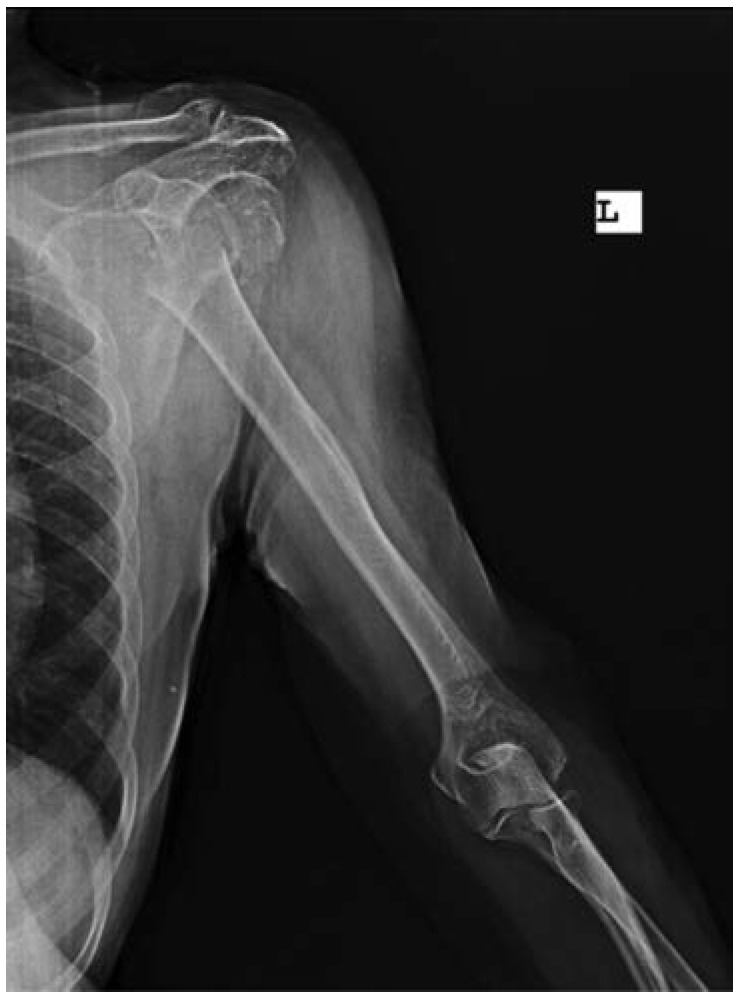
Radiographic image of the humeral head fractures and dislocation.
